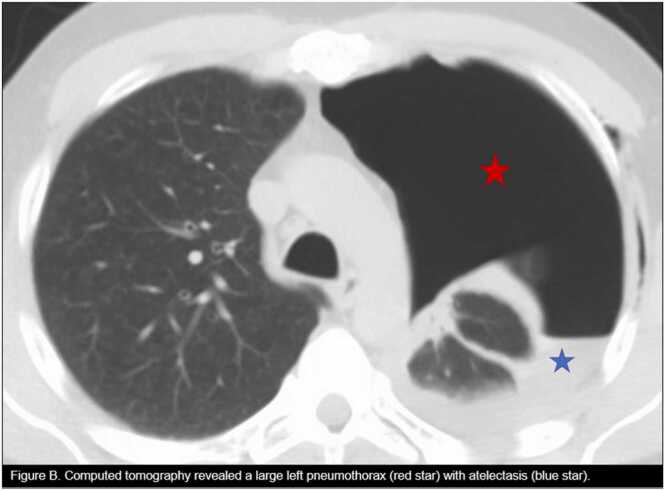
Computed tomography revealed a large left pneumothorax (red star) with atelectasis (blue star).
